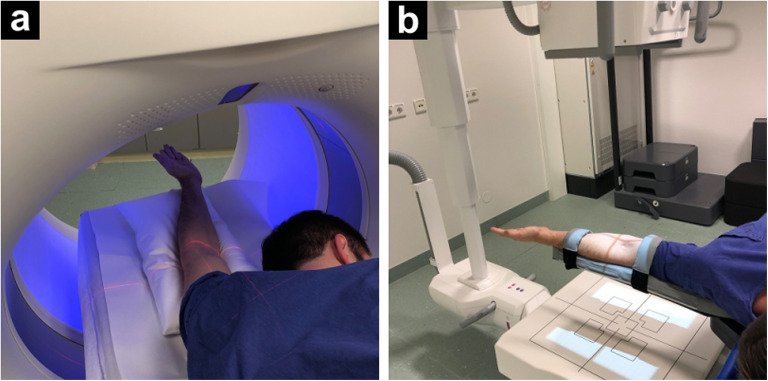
Positioning for image acquisition using a contemporary high-end multidetector computed tomography scanner (a) according to the modified “superman” position, and the twin robotic x-ray system cone-beam computed tomography scan mode (b) with the elbow positioned besides the patient table
